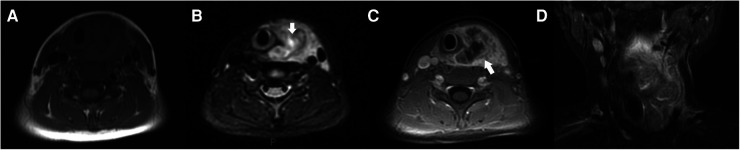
(A–D) A boy aged 8 years and 10 months had a left neck mass with a fever for 5 days. (A) T1WI and (B) T2WI STAIR: The mass in the left anterior region of the neck showed iso-and hypo-intensity on T1WI and hyper-intensity on T2WI with the unclear boundary and the necrotic area (arrow); the adjacent trachea and carotid sheath are pushed, and the left lobe of the thyroid is not clearly displayed. (C) The contrast-enhanced MRI showed inhomogeneous thick-walled enhancement of the mass in the left anterior cervical region (arrow) with the abscess and involvement of the left lobe of the thyroid. (D) The coronal contrast-enhanced MRI distinctly showed the extent of lesion involvement.
